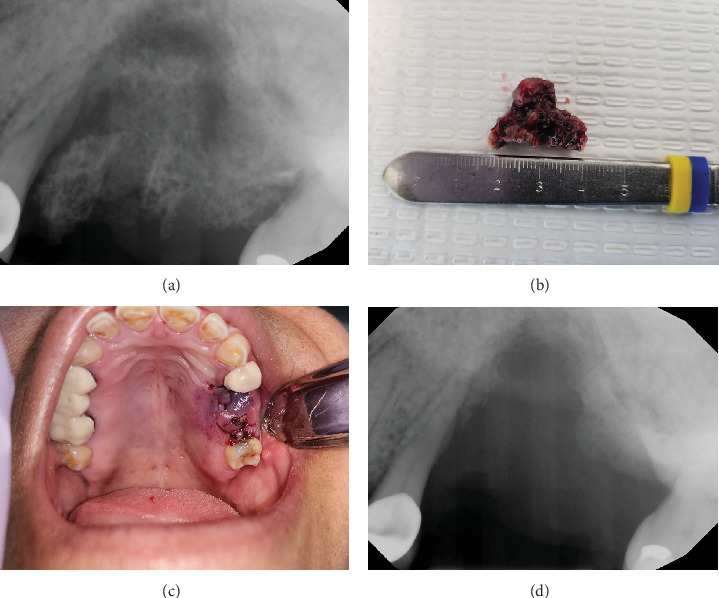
A 59-year-old female with medication-related osteonecrosis of the jaw. (a) A periapical radiograph showing a radiolucent rim separating the sequestrum from the healthy bone. (b,c) A sequestrectomy was performed, and the sequestrum measured 22 mm × 12 mm in size. (d) Postsequestrectomy periapical radiograph.
